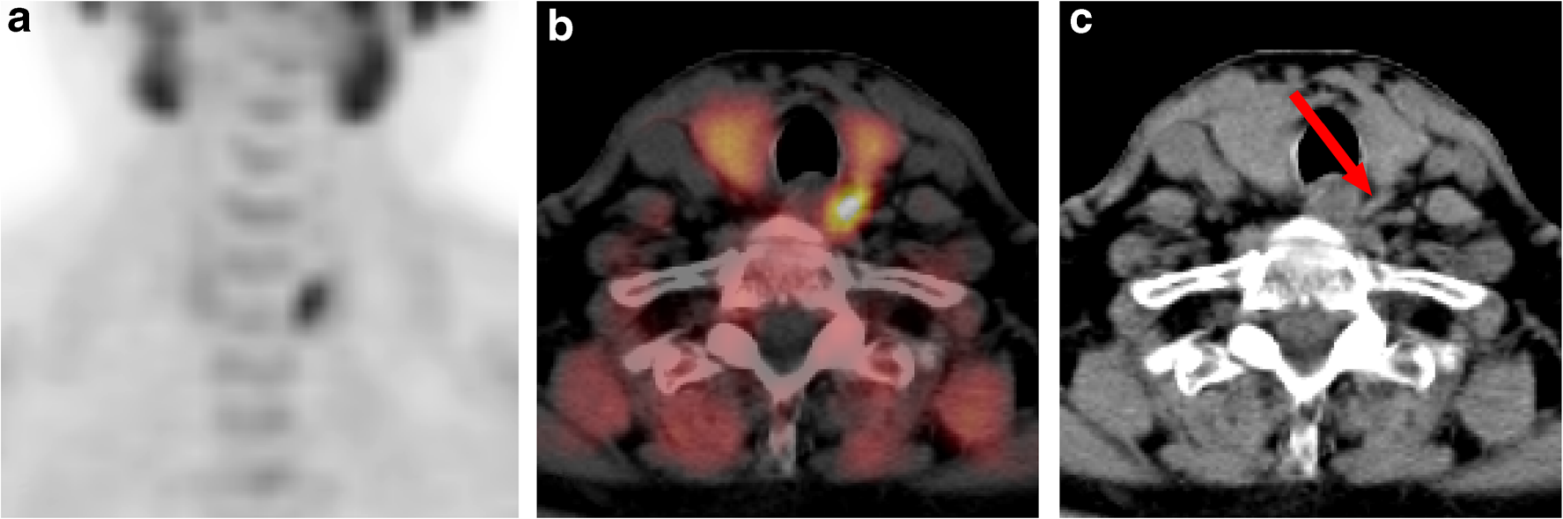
FCH PET/CT images of a 66-y-old male patient with PHPT and negative cervical ultrasound and MIBI SPECT/CT. (a) FCH PET maximum-intensity-projection, (b) FCH PET/CT fusion transverse slice, and (c) low-dose CT transverse slice, showing increased FCH uptake in a flat PTA in the left superior position (red arrow). Resection of a flat left superior PTA was performed by minimally invasive parathyroidectomy, leading to cure
